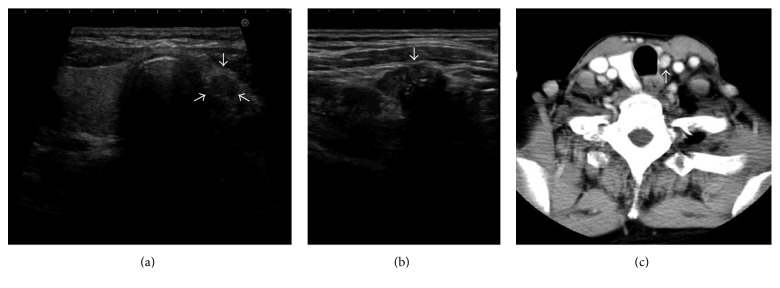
Findings from ultrasonography and computed tomography. The right thyroid lobe and thyroid isthmus are present, while the left lobe of the thyroid is absent. A nodule measuring 0.7 × 0.8 × 1.4 cm is seen on the left side of the thyroid isthmus. On cervical ultrasonography, the periphery of the tumor is nonuniform, small calcifications are detected in the interior, and the posterior echo is attenuated. Arrows indicate the tumor. No cervical lymph node swelling is detected.
